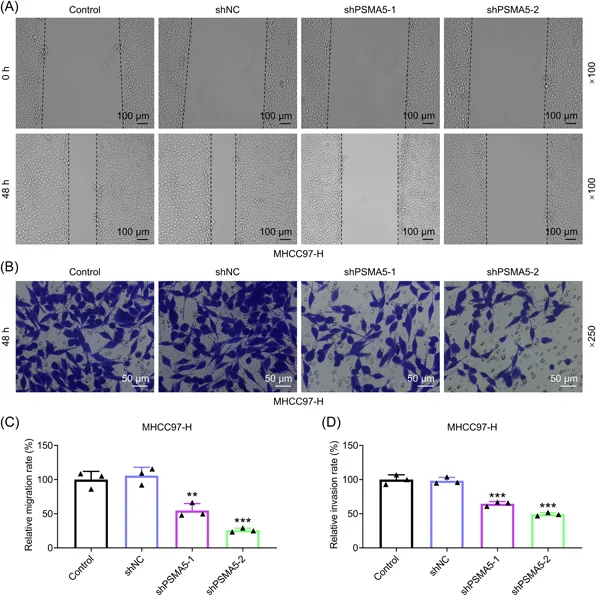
PSMA5 knockdown inhibited the migration and invasion of HCC cells. (A, C) The migration of MHCC97‐H cells transfected with shNC or shPSMA5‐1/−2 was evaluated by wound healing assay (magnification: ×100; scale: 100 µm). (B, D). The invasion of MHCC97‐H cells transfected with shNC or shPSMA5‐1/−2 was evaluated by Transwell assay (magnification: ×250; scale: 50 µm). *p* < .01; p* < .001; * versus shNC. HCC, hepatocellular carcinoma; PSMA5, proteasome subunit alpha 5; shNC, short hairpin RNA against negative control; shPSMA5, short hairpin RNA against PSMA5.
